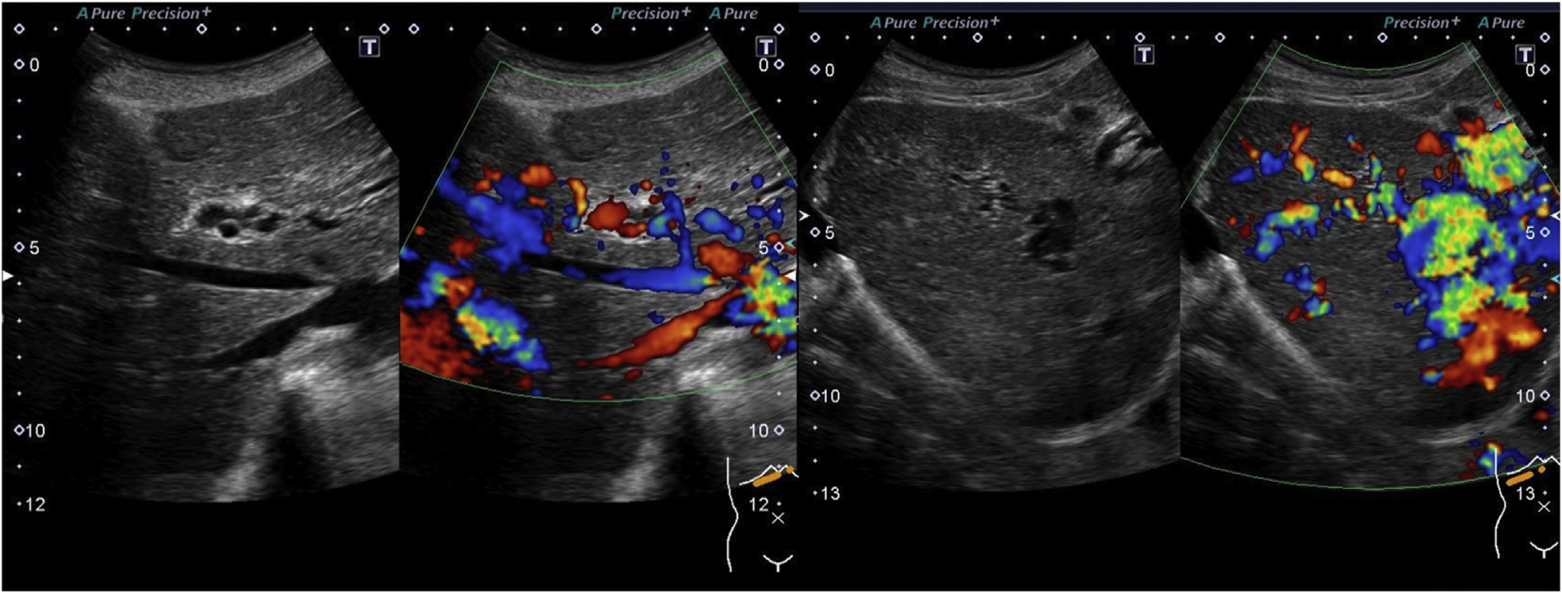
Ultrasound of the Liver Ultrasound represented abundant intrahepatic vessels.
